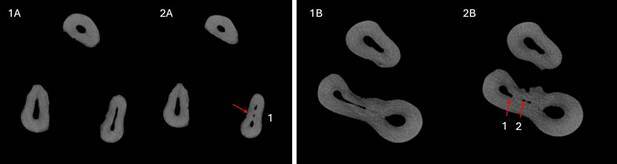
Axial sections of micro-computed tomography of upper primary first molars showing the root canal system of two different teeth from the sample. Images 1A and 2A correspond to different axial sections of the same sample, while images 1B and 2B correspond to different axial sections of another sample. Some accessory canals are indicated by red arrows. In A, the arrow demonstrates one accessory canal (1A and 2A); in B, the arrows indicate two accessory canals (1B and 2B).
